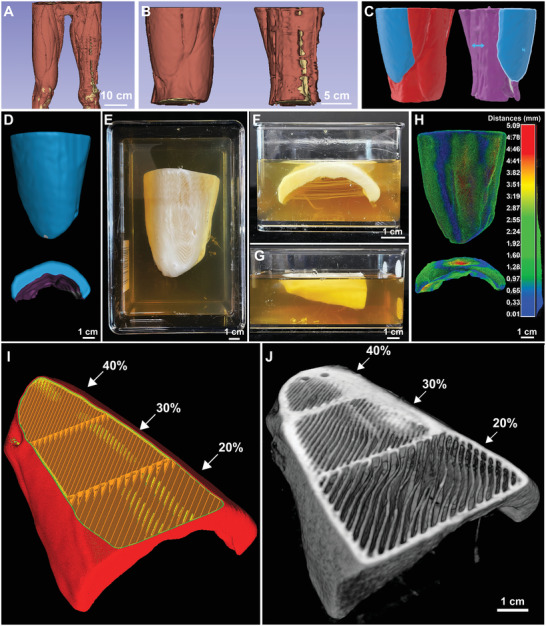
Patient‐specific dECM scaffold with spatial control for implantation into a human VML injury. A) Image segmentation was utilized to create the model, and B) the model was cropped to a smaller section above the knee. C) The scaffold was created by isolating the vastus lateralis muscle from the uninjured leg and overlaying it onto the injured top of the isolated injured leg model‐aligning the bone and outer section. D) A “subtraction” Boolean modifier was then used to create a model from the difference between the isolated healthy muscle and the injured leg. The model was then imported into MeshMixer to cut out the center section and smooth the rough edges. This model was then sliced to generate the G‐Code and printed with 1:1 ratio of collagen type 1: UBM with 2% barium sulfate. E‐G) Photographs of the printed scaffolds from top and side views. H) Dimensional gauging analysis of the 3D printed scaffold to the original CAD model. I) The infill angle was modified to be 45° to match the innate muscle fiber alignment of the vastus lateralis muscle, and infill density was varied from 20%–40%. J) 3D visualization from a CT scan of structure with an exposed slice to show the varying infill percentages and 45° fiber alignment to match the muscle alignment of the vastus lateralis muscle.
